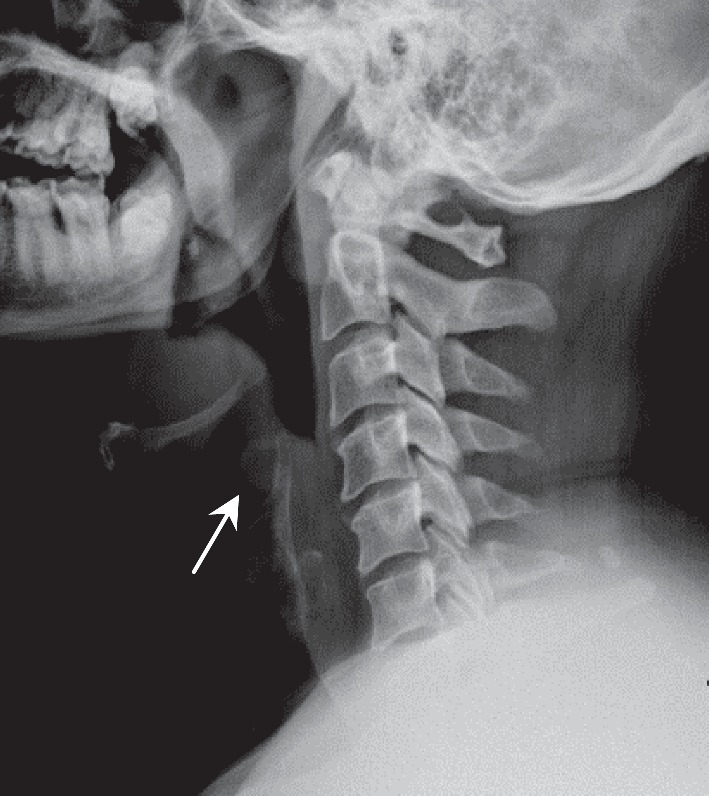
Radiography of lateral soft tissue neck.
